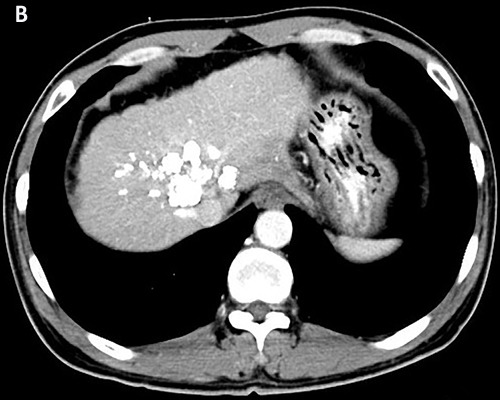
Tumor shrinkage was confirmed CT scan on March 2016 A. and on May 2016 B. showed that tumor was smaller after using apatinib.
